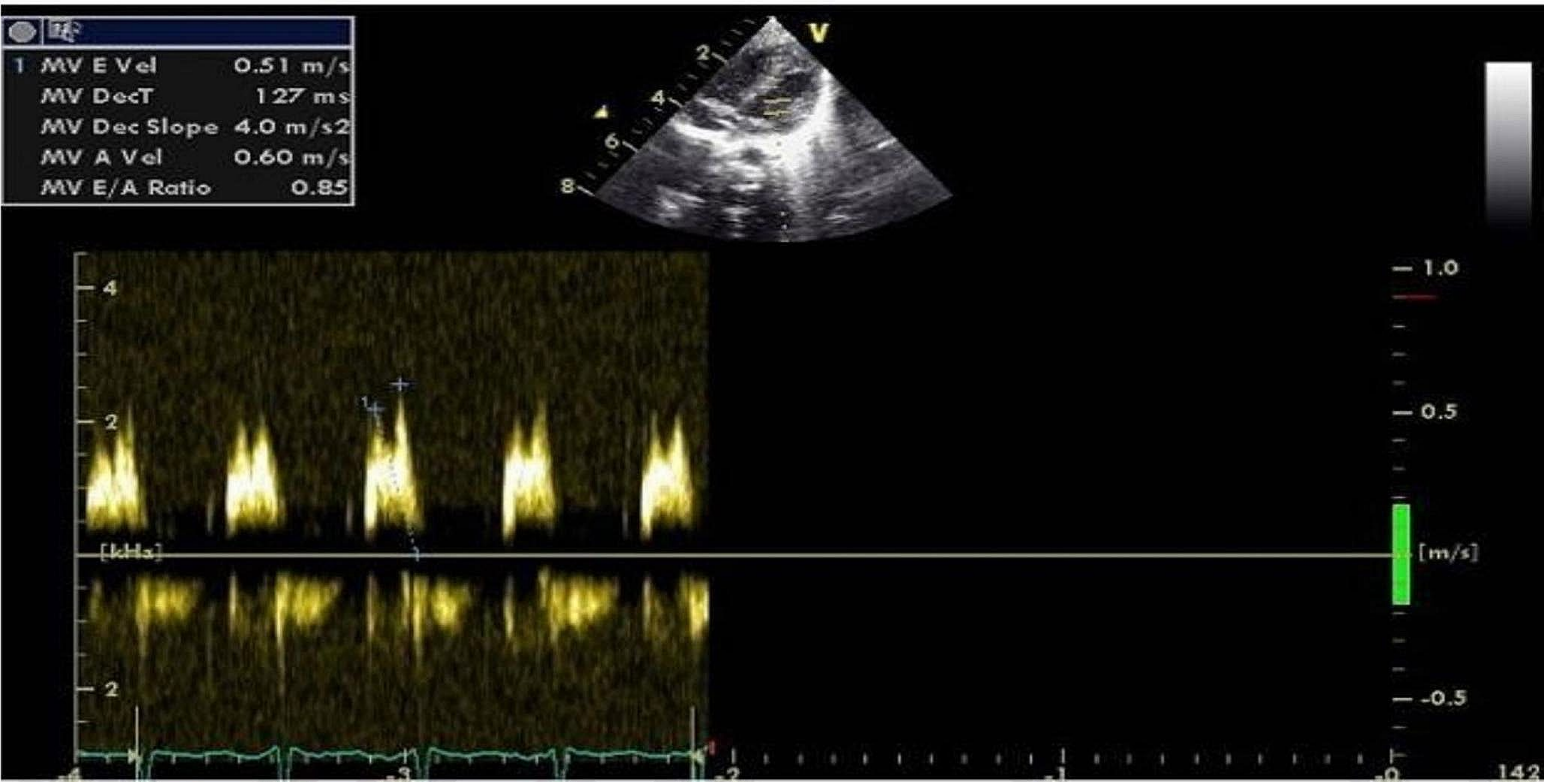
LV diastolic function by conventional doppler
